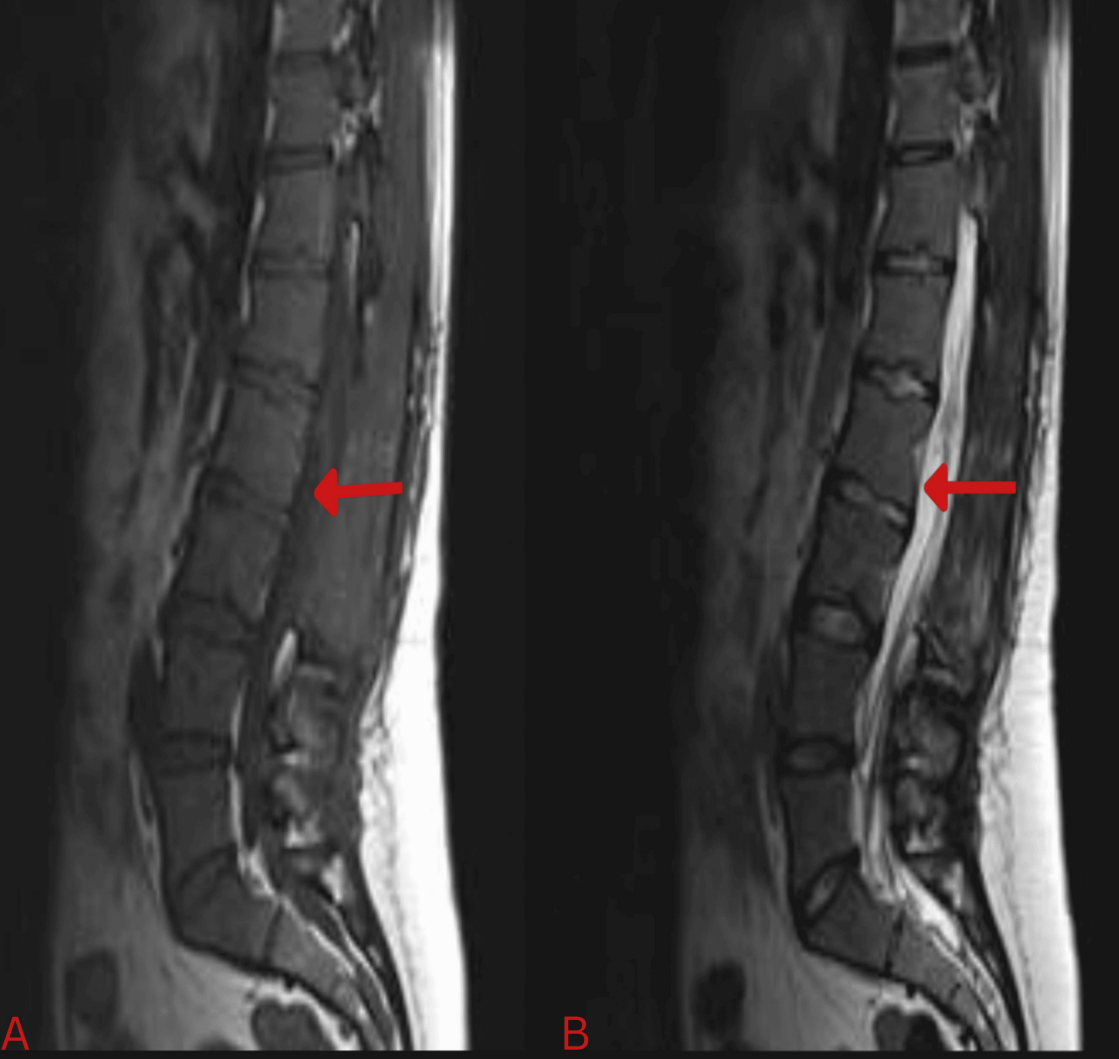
Postoperative sagittal T1-weighted (A) and T2-weighted (B) contrast-enhanced MRI of the lumbosacral spine showing complete removal of the lesion. MRI, magnetic resonance imaging
